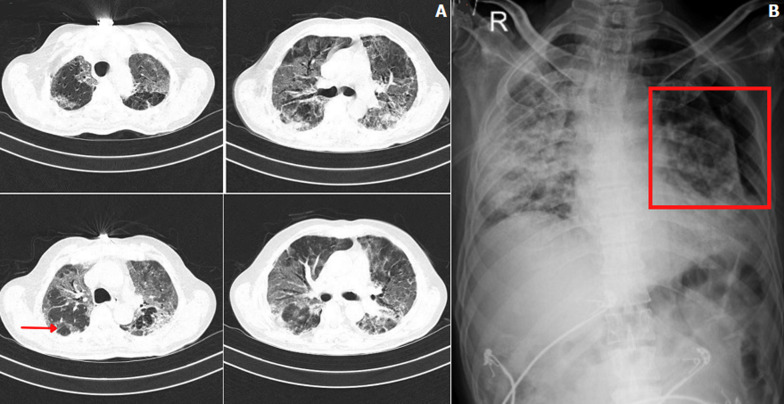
(A) HRCT thorax showing multiple air-filled cystic spaces (red arrow) in sub-pleural spaces of lateral segment of right middle and lower lobes with CT severity score 23/25; (B) chest X-ray showing secondary spontaneous pneumothorax
